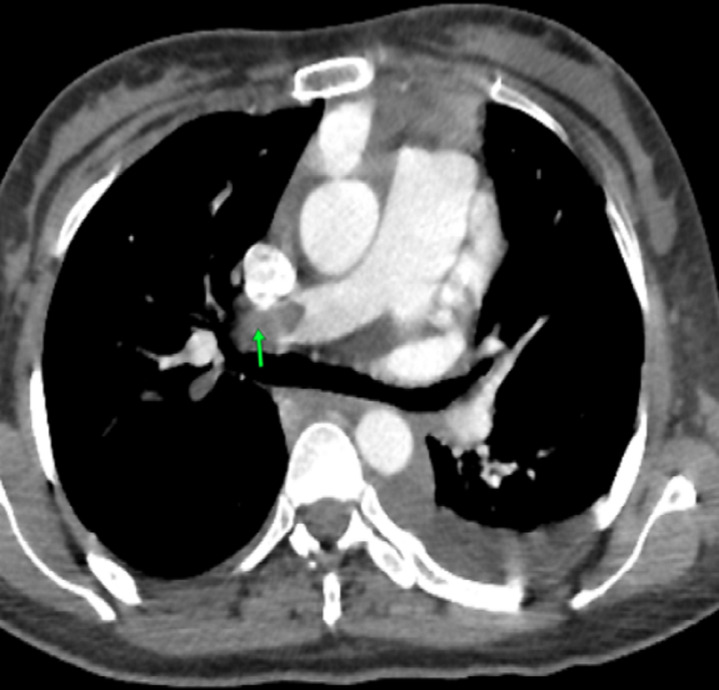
axial computed tomography pulmonary angiogram image showing pulmonary embolism of the right pulmonary artery
